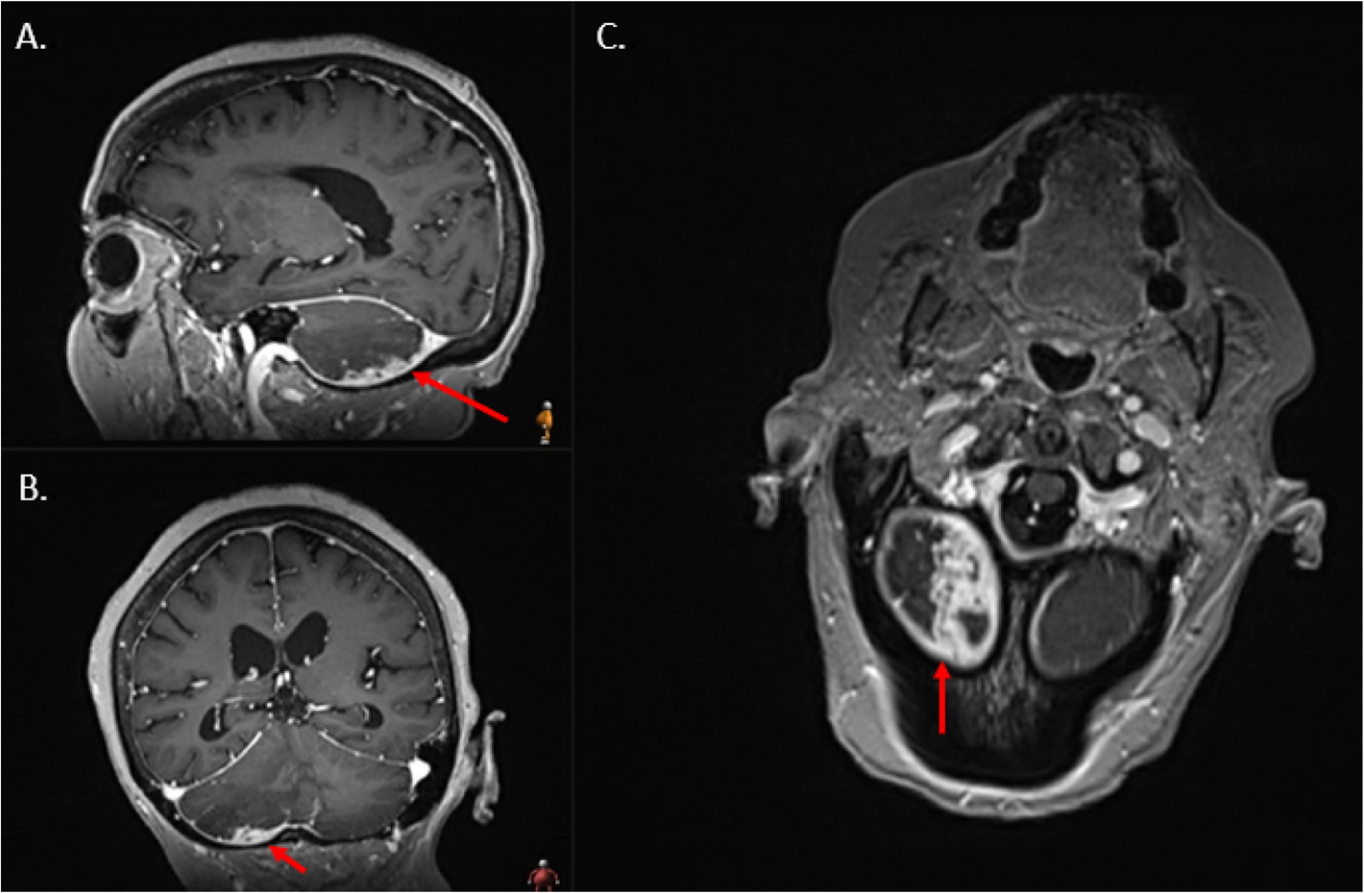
T1-weighted MRI with contrast demonstrates a right dural-based enhancing mass (red arrows) within the posterior fossa along the inferior border of the right cerebellar hemisphere on a. sagittal b. coronal and c. axial reformatted images
